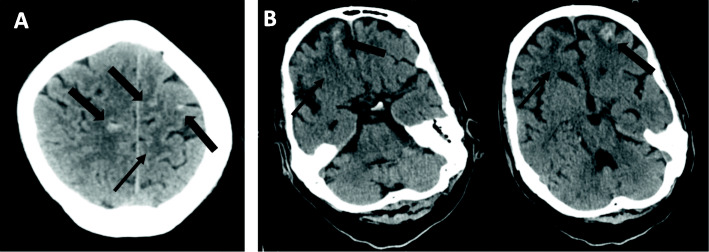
Exemplary cranial CT scans with diffuse sulcal/subarachnoidal bleedings frontally, frontobasally and parietally (wide arrows), and partly confluent frontobasal and subcortical hypodensities (slim arrows) indicating infarction in a a 48 year-old male (patient #39) and b a 56 year-old male (patient #37) with both severe COVID-19 and acute respiratory distress syndrome
